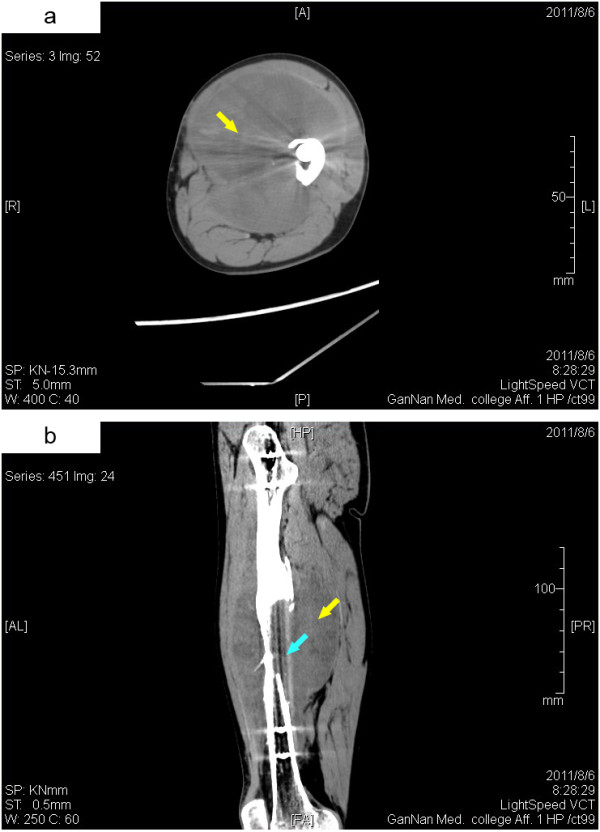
CT images of the left thigh. (a) Transverse image. (b) Coronal image. A high-resolution volumetric CT scanning revealed a 9.7 × 11 × 19 cm irregularly shaped soft tissue mass in the anteromedial left thigh (yellow arrow) and a partial bone defect approximately 9.7 cm in length on the medial border of the left femur shaft (light blue arrow).
